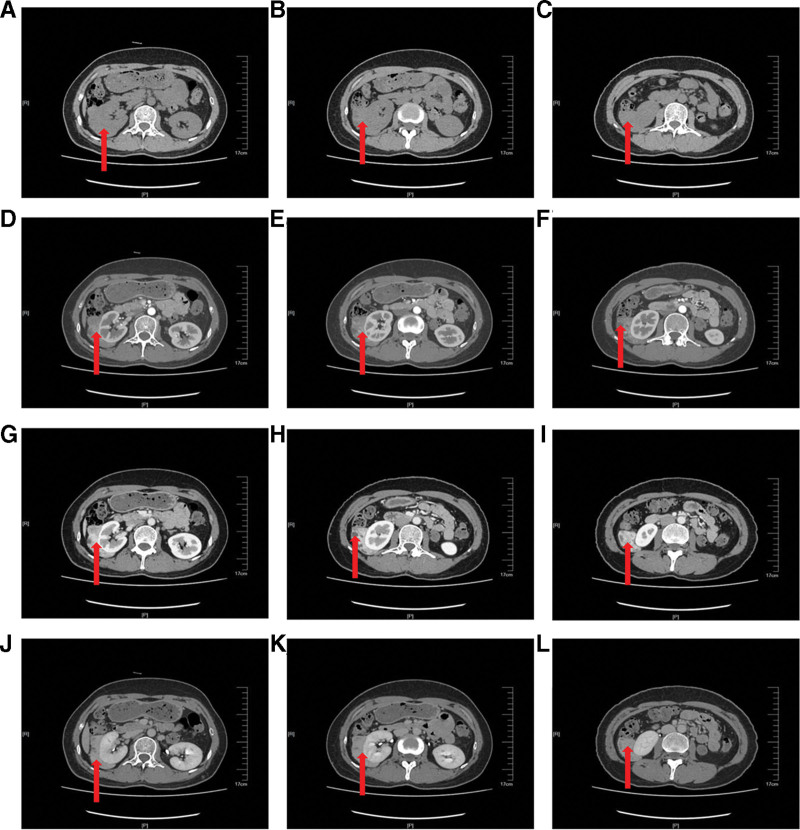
Preoperative renal CT reveals a large extrarenal mass, suggestive of a fat-poor AML. AML = angiomyolipoma, CT = computed tomography.
